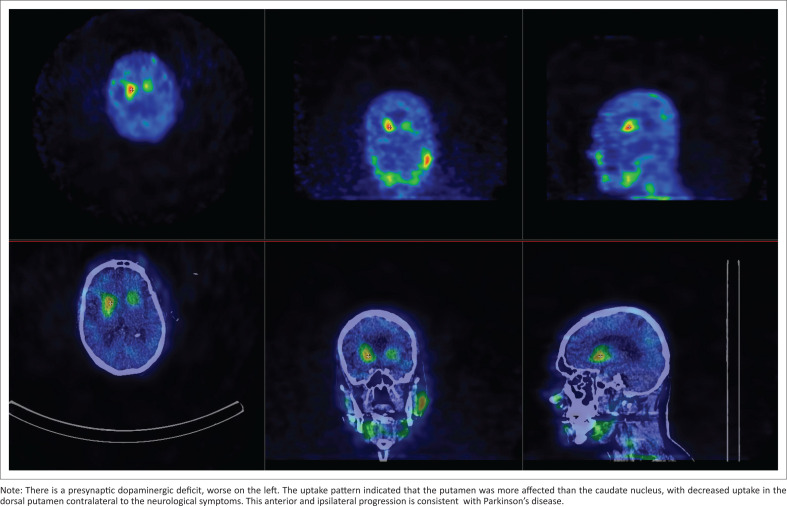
Brain Single Photon Emission Computed Tomography (SPECT).
